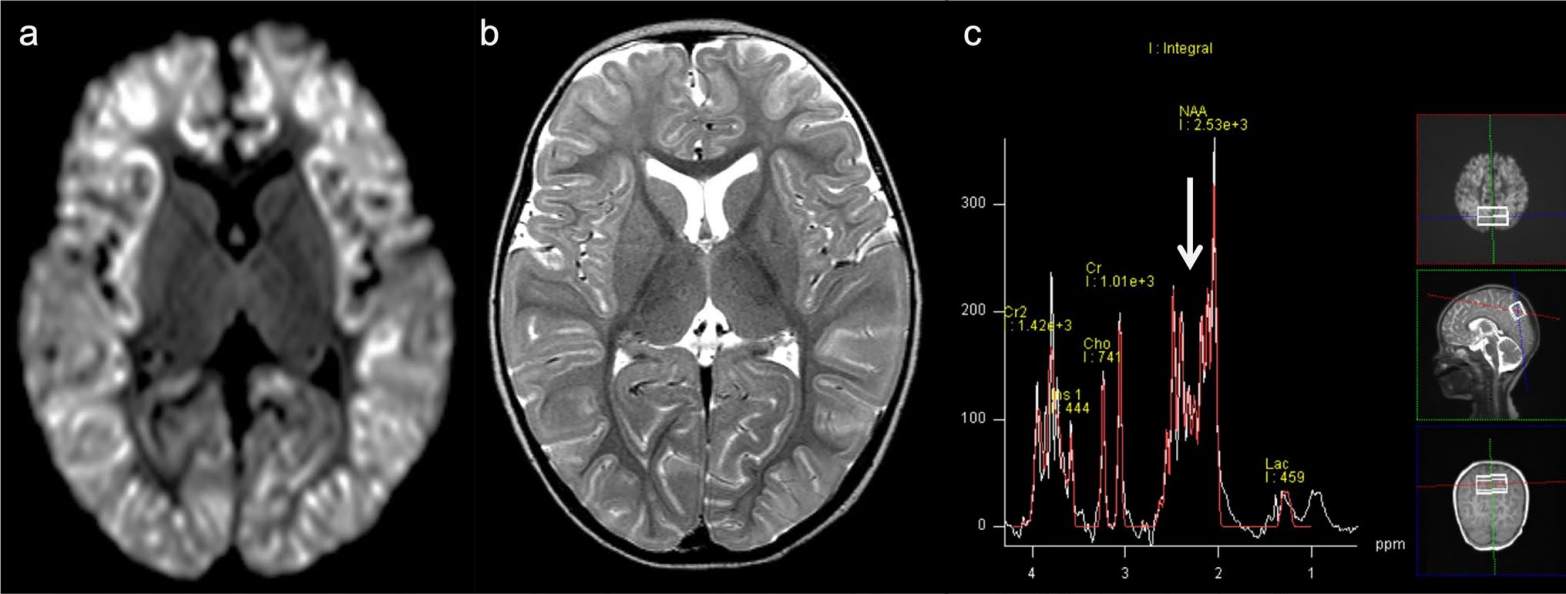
Ornithine transcarbamylase (OTC) deficiency. A 2-year-old, female patient with acute hyperammonemic encephalopathy. a, b DWI (a) and T2-weighted images (b) demonstrated a diffuse, scalloped ribbon pattern of injury to the cerebral cortices. c ^1^H-MRS (TE = 30 ms) of the affected bilateral parietal lobes indicated a slight elevation of Glx (arrow)
